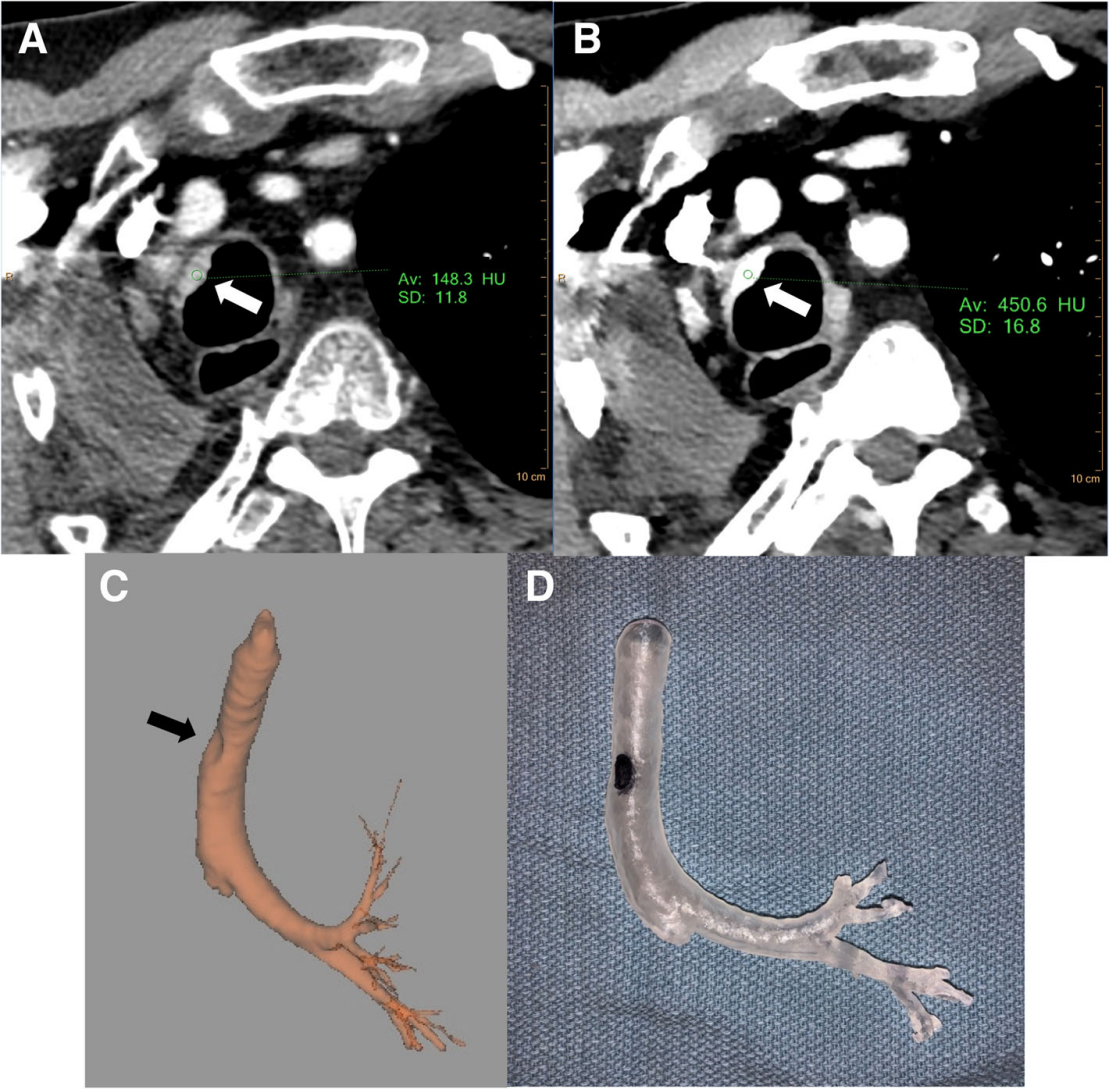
Conventional (a) and VMI 40 keV (b) axial SDCT images of case 3 malignant tracheal lesion. The recurrent tracheal malignancy (white arrow) is enhanced in the 40 keV VMI compared to conventional CT. 3D volume rendering and segmentation of the bronchial tree from the 40 keV VMI data (c) also demonstrates good definition of the tracheal lesion (black arrow). (d) 3D printed bronchial tree from the 40 keV VMI data at 50% scale. The Formlabs Form 2 with standard clear resin was used and the tracheal lesion indentation was marked with black ink for visualization
